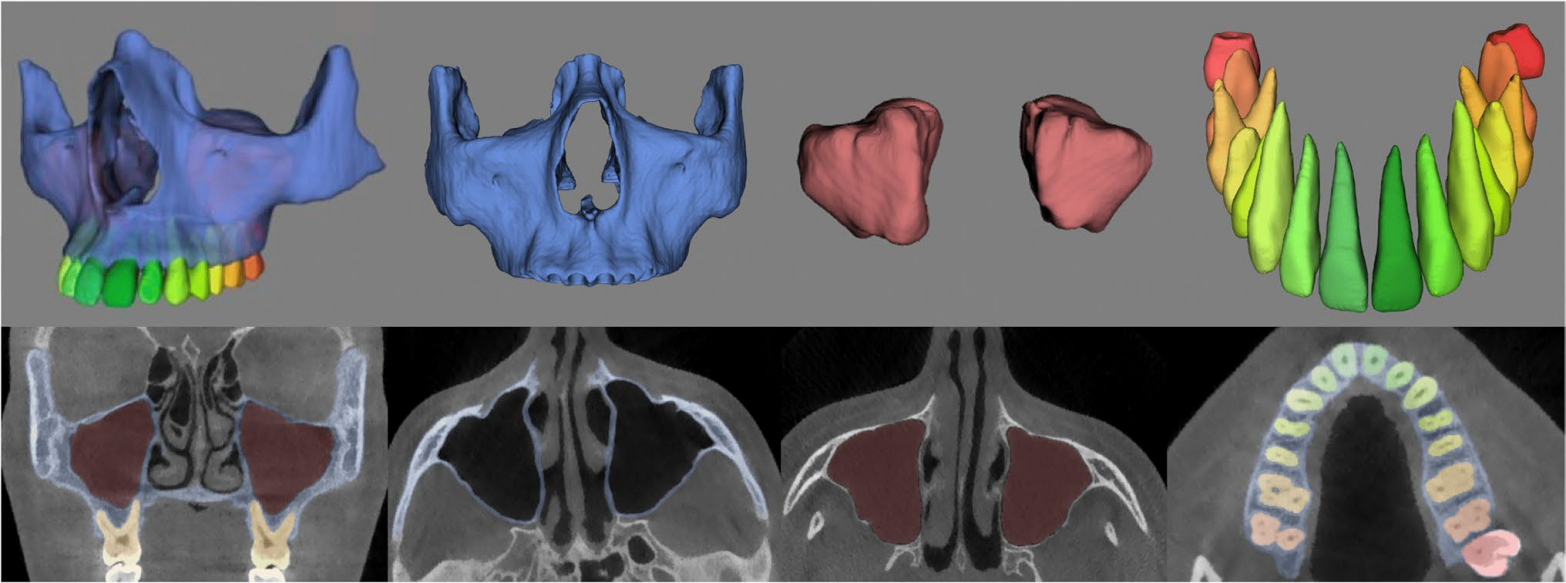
3D views and their respective colored segmentations on CBCT slices on Virtual Patient Creator (creator.relu.eu, Relu BV, Version December 2021): a all structures combined showing the maxillary virtual patient, b maxillofacial complex, c maxillary sinuses, and d upper dentition
